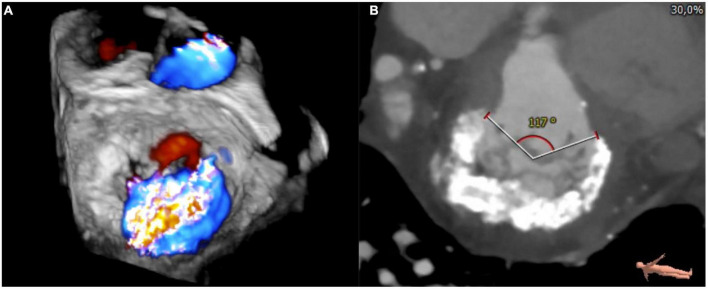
3D transesophageal echocardiography (A) and cardiac computed tomography (CT)-scan (B) showing severe mitral annular calcification (MAC) and coexisting severe mitral regurgitation.
